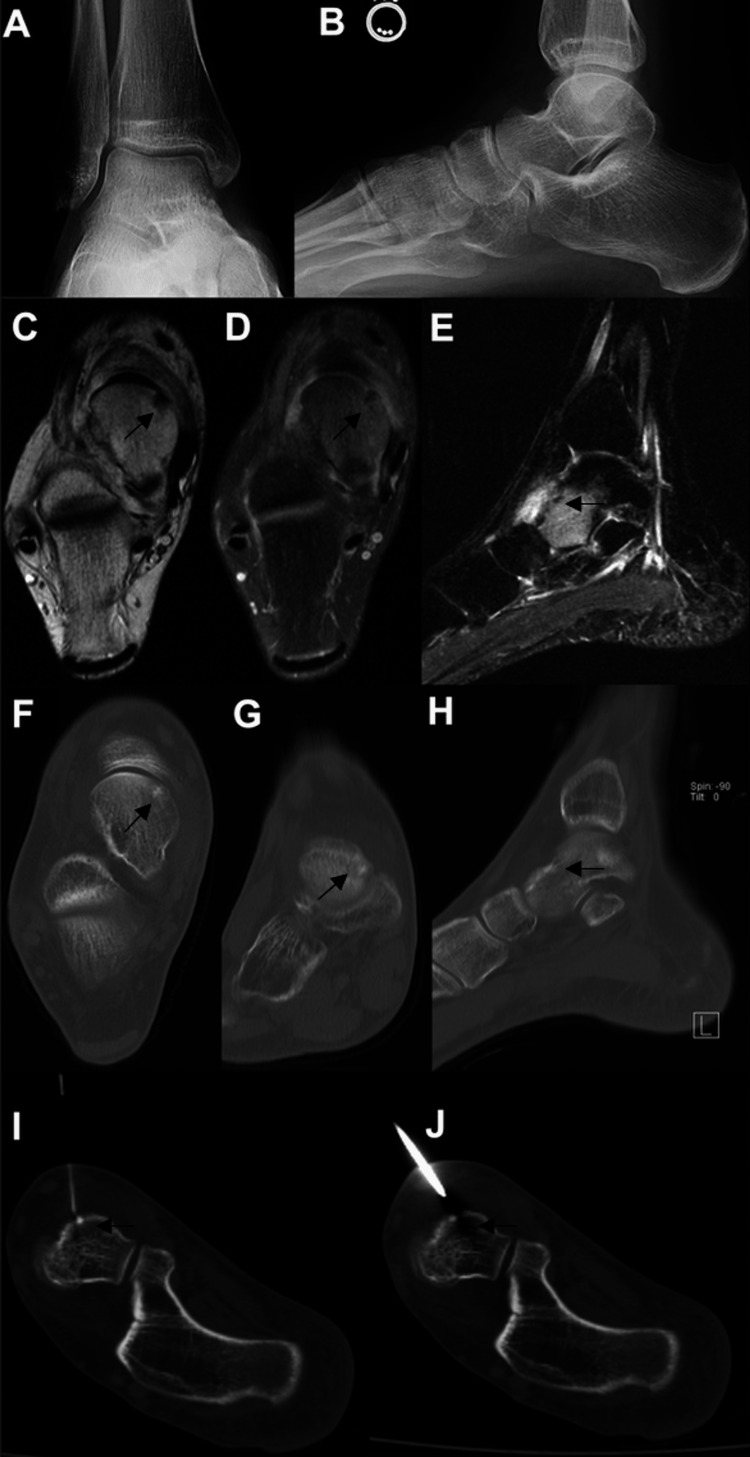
Images of the patient discussed in case two. Initial AP (A) and lateral (B) weight-bearing radiographs of the patient in case two were normal. The osteoid osteoma was identified on MRI and is highlighted by the lesion shown on the axial T1 (C) and fluid sensitive (D) sequences; extensive edema around the lesion was seen on the sagittal inversion recovery sequence (E). The nidus of the osteoid osteoma is seen on the CT axial (F), coronal (G), and sagittal (H) images. RFA localization of the lesion via CT is shown in I and J. MRI: magnetic resonance imaging; CT: computed tomography; RFA: radio-frequency ablation
